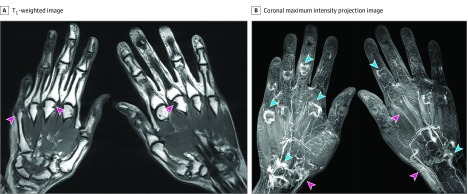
Magnetic Resonance Image of Bilateral Hands in an Individual With Thyroid Cancer With Inhibitor–Induced Inflammatory Arthritis (Patient 2) A, T1-weighted image of both hands reveals multiple marginal osseous erosions (pink arrows). B, Coronal maximum intensity projection image of both hands, generated from gadolinium contrast enhanced T1-weighted 3-dimensional gradient echo images, shows synovial enhancement at metacarpophalangeal, proximal interphalangeal and intercarpal joints (blue arrows), as well as tenosynovitis (pink arrows).
